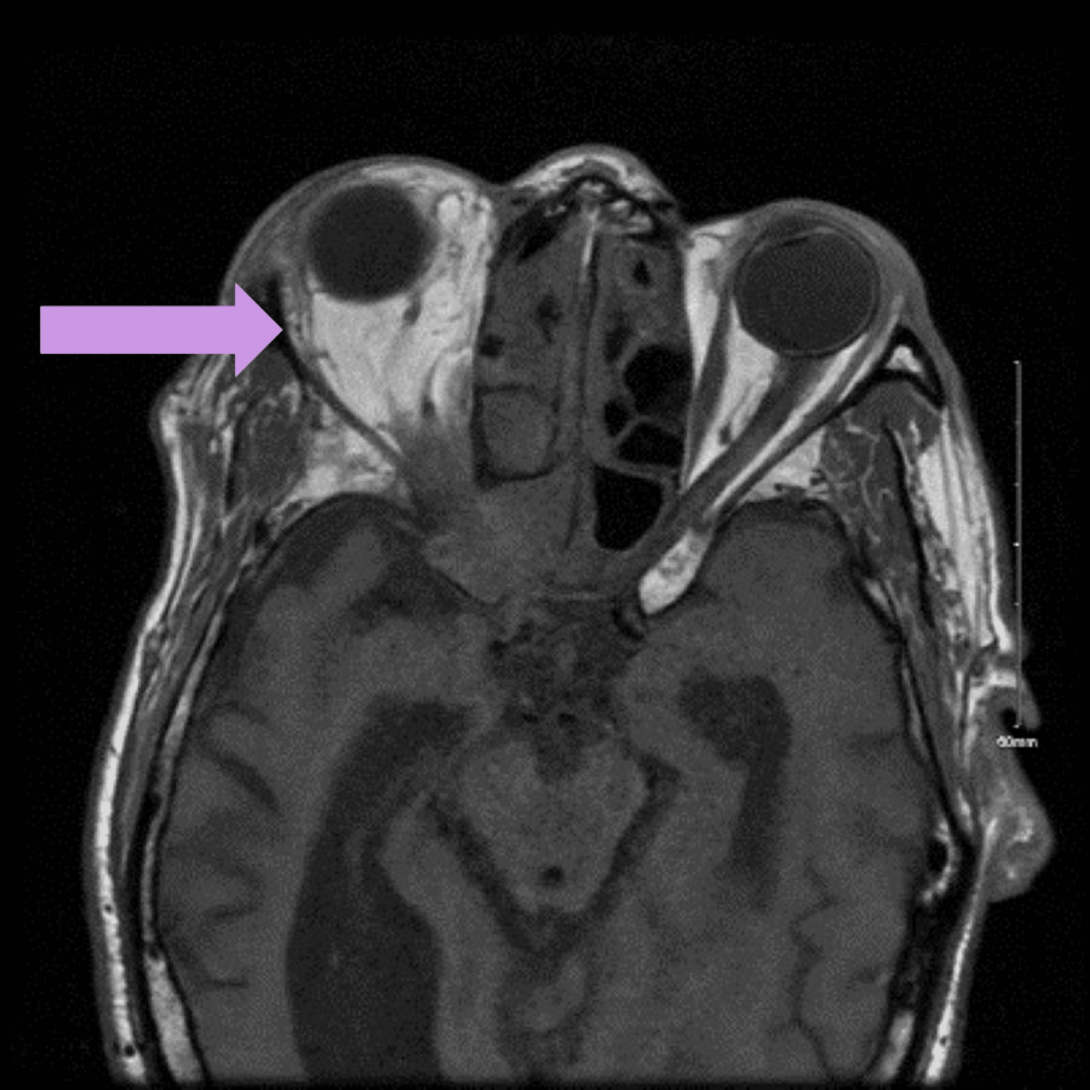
Magnetic resonance imaging of the brain and orbits showing ill-defined infiltrative enhancement centered at the orbital apex involving the right superior, medial, inferior, and lateral rectus muscles, the right orbital floor, anterior clinoid process, cavernous sinus, pterygoid process, masticator space, and temporal fossa.
